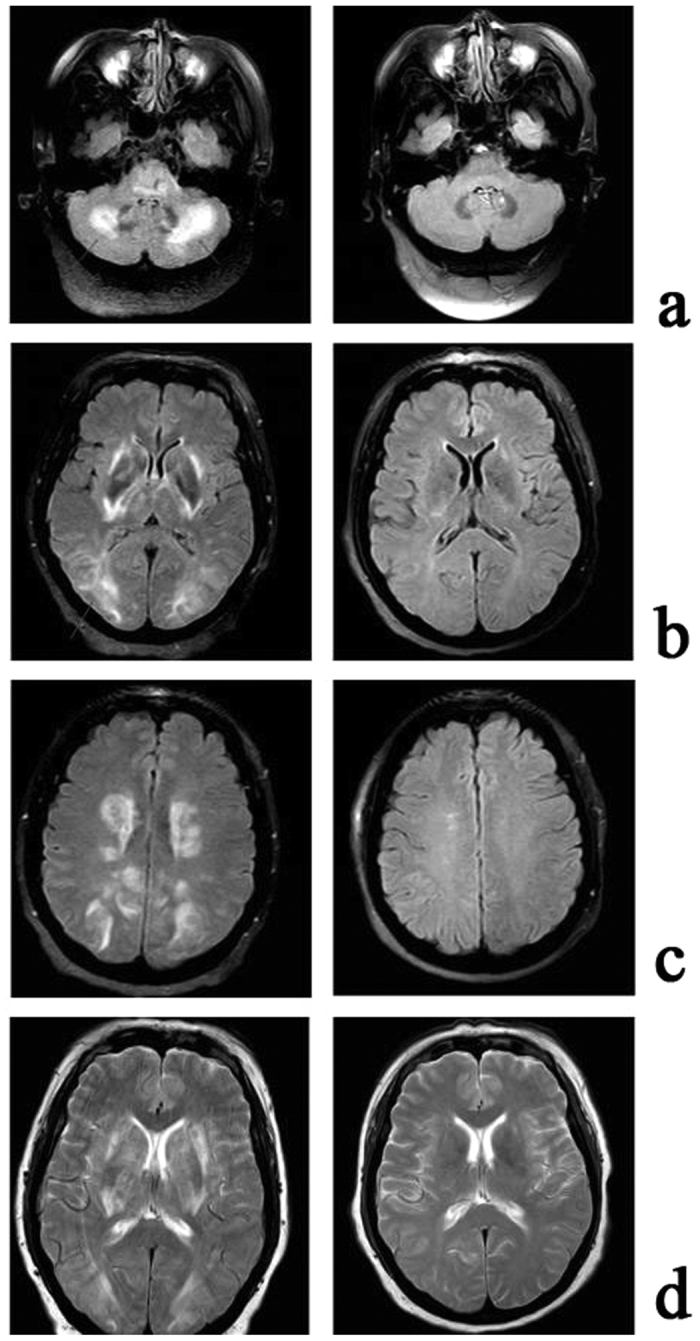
MRI of 51-year-old female CMS patient. Each panel shows acute (left) and recovery (right) images. Note markedly increased T_2_ signal in cerebellar hemispheres (a), internal capsule, parietal and occipital lobes (b–d). Abnormal signal disappeared two months later, with the patient fully recovered.
